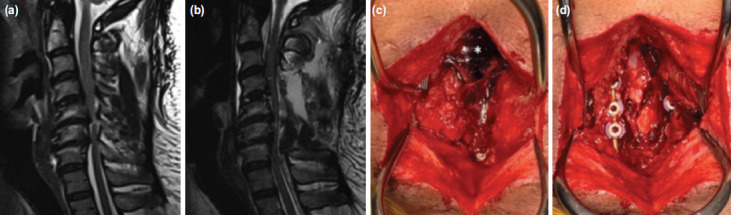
Case 2; (a) MRI showing C4/5, C5/6 and C6/7 spinal stenosis with spinal cord oedema. (b) MRI showing spinal epidural haematoma with spinal cord compression; and (c) intra-operative photo showing spinal epidural haematoma (white asterisk) and (d) complete decompression of the spinal cord after evacuation of haematoma.
